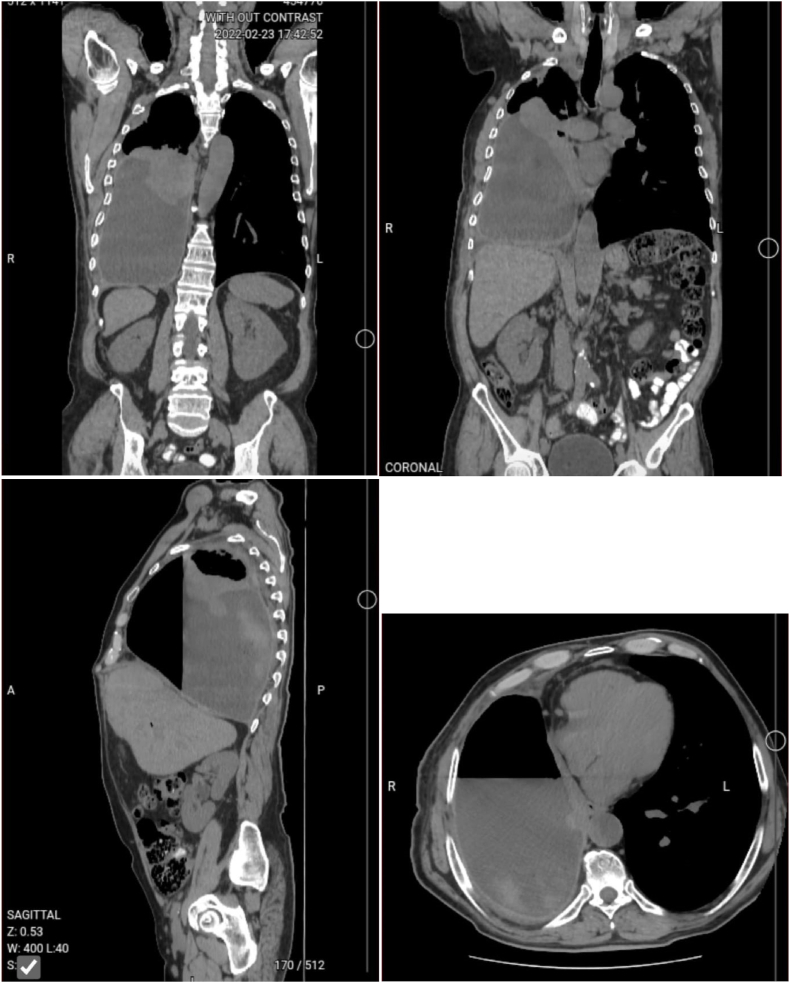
CT scan of the chest showing: Large right side pleural effusion causing compression atelectasis and loss of volume of right lung.
